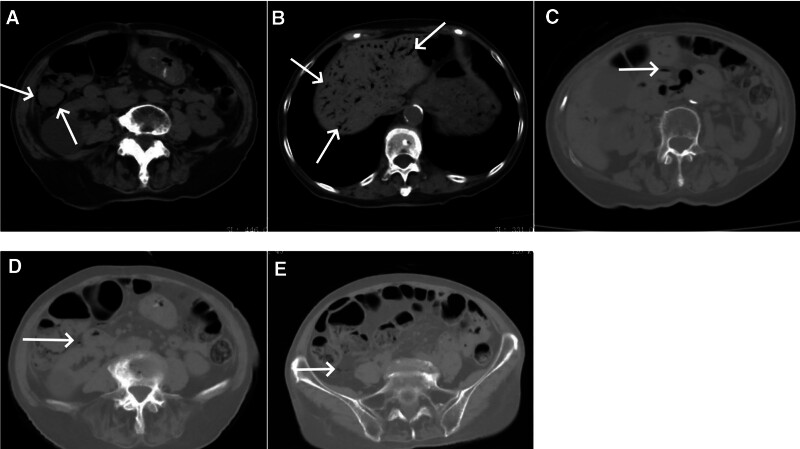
Abdominal computed tomography (CT) conducted during the onset of severe abdominal pain on the 5th day after operation. (A) Thickening of the colonic hepatic flexure wall with densely clustered small cystic lucencies (arrows) within the wall. (B) Abundance of branching lucencies (arrows) within the liver along the portal vein distribution, gradually tapering from the porta hepatis toward the periphery, presenting a “dead tree branch” appearance. (C) Presence of gas within the superior mesenteric vein (arrow). (D) Minimal gas accumulation in the right colonic vein (arrow). (E) Slight gas accumulation in the ileocolic vein (arrow).
